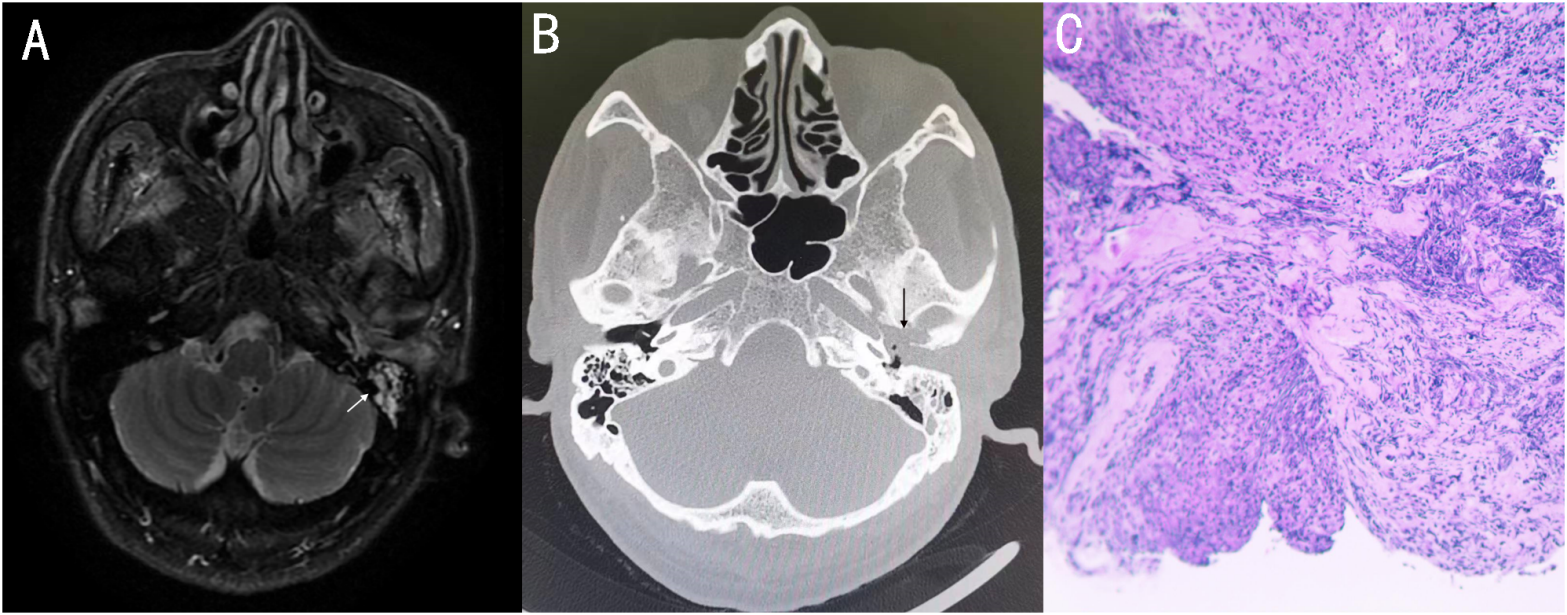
(A) MRI showed thickening and strengthening of the wall of the left external auditory canal cavity and left mastoiditis. (B) Axial CT showing soft tissue involvement of the left external and the middle ear, with external auditory canal - tympanum wall bone erosion. (C) 11-10 Postoperative pathology: granulation tissue proliferation with extensive neutrophil infiltration.
